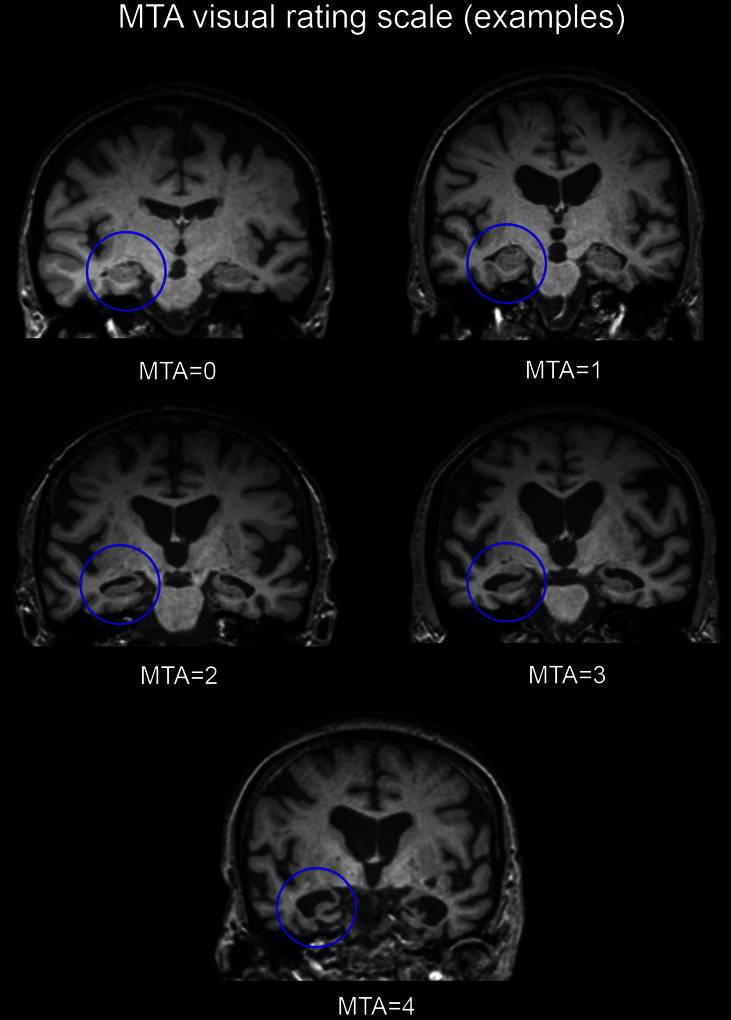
Coronal T1-weighted images depicting bilateral atrophy at each level of the MTA five-point scale.
